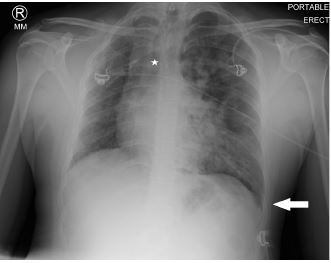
An anteroposterior plain film of the chest revealing a deviated trachea (star) and a deep sulcus sign (arrow), in addition to fluffy infiltrates in the left lung fields.
